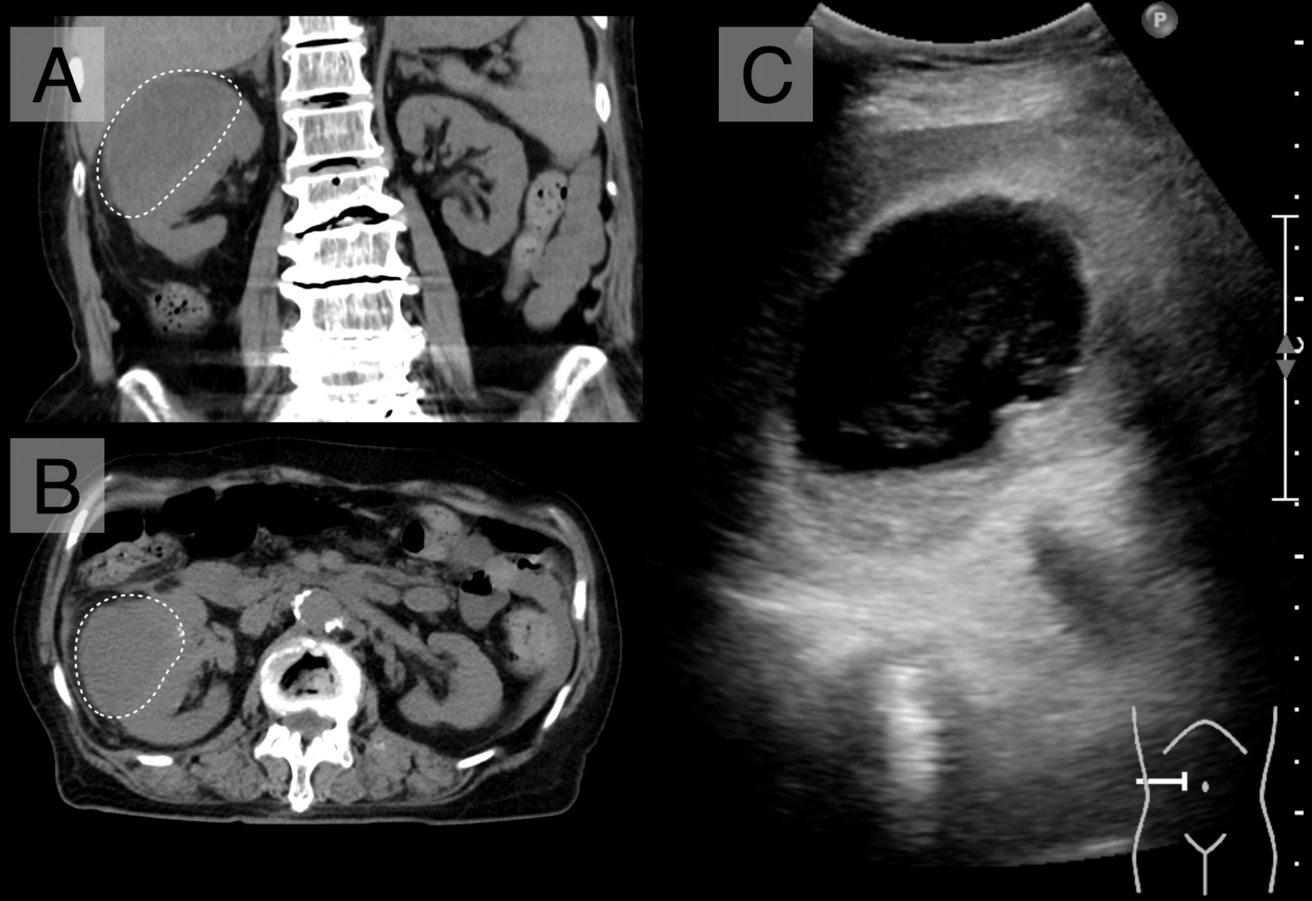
Imaging findings on admission. A non-contrast CT showed an enlarged right renal cyst (75 × 55 × 38 mm, dotted circles) with increased density and wall thickening accompanied by pericystic fat stranding. (A) Coronal and (B) axial views. (C) Abdominal ultrasonography showing debris-like internal echoes.
